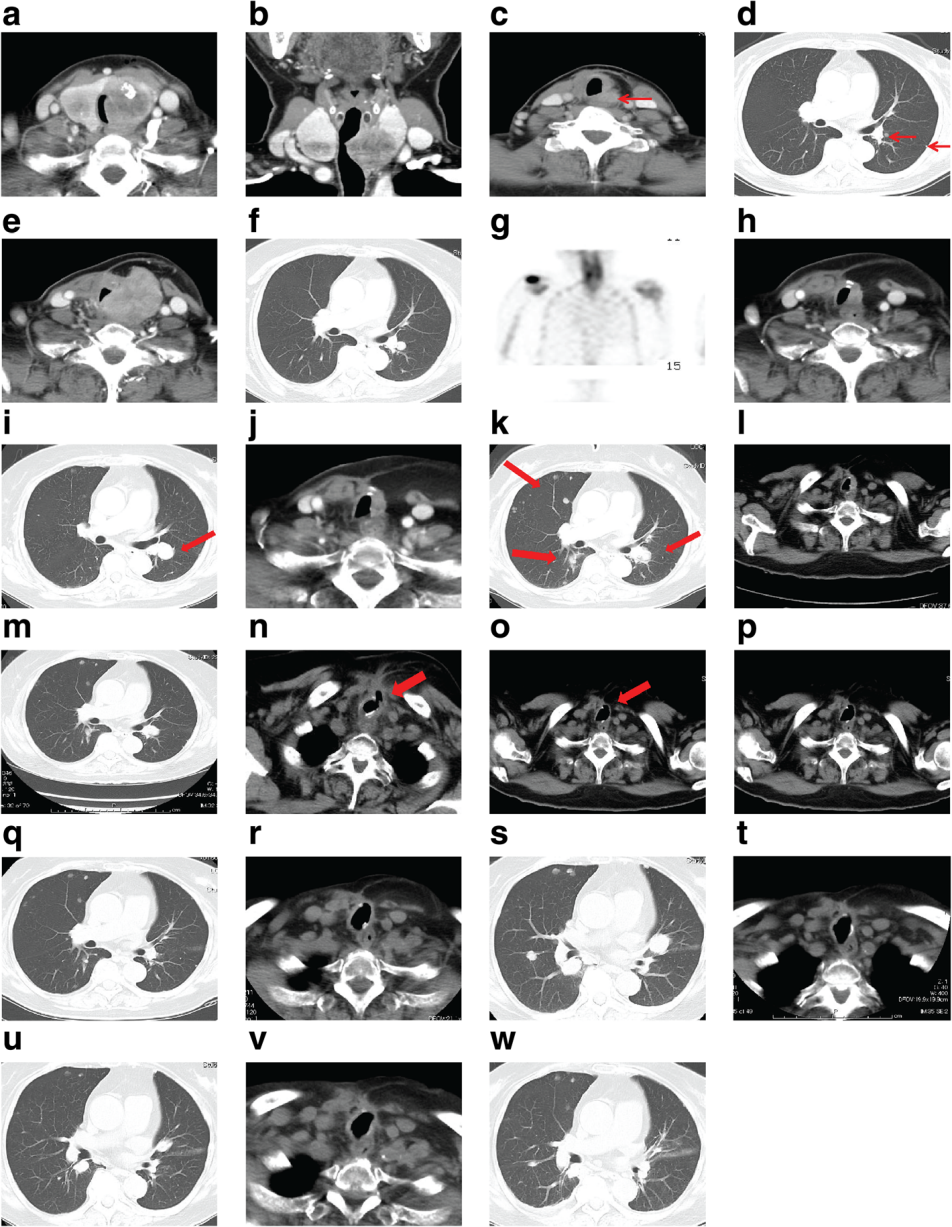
a and b Enhanced CT findings before operation. The tumor (arrow) was mainly located in the left lobe and invaded into half the tracheal circumference (40 × 36 mm) (Fig.2(A)). c and d Recurrent tumor was found just below the left piriform fossa (15 × 11 mm) (c), and lung metastasis (max, 6 mm) (d) was found at the same time 3 months after the operation by CT scan (Fig.2(B)). e, f, and g Nine months after the operation, CT scan showed prominent tumor progression in the neck (48 × 38 mm) (e) and the lung (18 × 16 mm) (f). Additionally, bone scintigraphy demonstrated a solitary bone metastasis in the right scapula (g) (Fig.2(C)). h and i Three years after the operation, local recurrence in the neck was controlled (15 × 11 mm) (h), although multiple metastasis in the lung worsened (PD). Maximum size was 30 × 26 mm (i) (Fig.2(D)). j and k Within 8 months after starting sorafenib, local recurrence (j) and lung metastasis worsened (PD) (k) (Fig.2(E)). l and m Within 1 month after starting lenvatinib, the tumor in the neck remained controlled (l) and multiple metastases in the lung decreased and diminished in size (18 × 15 mm) (m) (PR) (Fig.2(F)). n Within 3 months after starting lenvatinib, pin-hole perforation (5 mm) of the trachea suddenly appeared at the end of tracheal invasion (Fig.2(G)). o Within 1 month after terminating lenvatinib, the perforation was naturally cured and pin-hole closed (Fig.2(H)). p and q Within 2 months after restarting lenvatinib, tumors in the neck (p) and the lung (q) were controlled (PR) (Fig.2(I)). r and sAlthough local recurrence (r) was kept controlled, lung metastasis (s) was exacerbated (21 × 18 mm) for 2 months after terminating lenvatinib because of adverse events (PD) (Fig.2(J)). tand uWithin 3 months after restarting lenvatinib, local recurrence in the neck (t) and lung metastasis (u) remained under control (PR) (Fig.2(K)). v and w One year and 9 months after starting lenvatinib, CT scan still showed PR (Fig.2(L))
